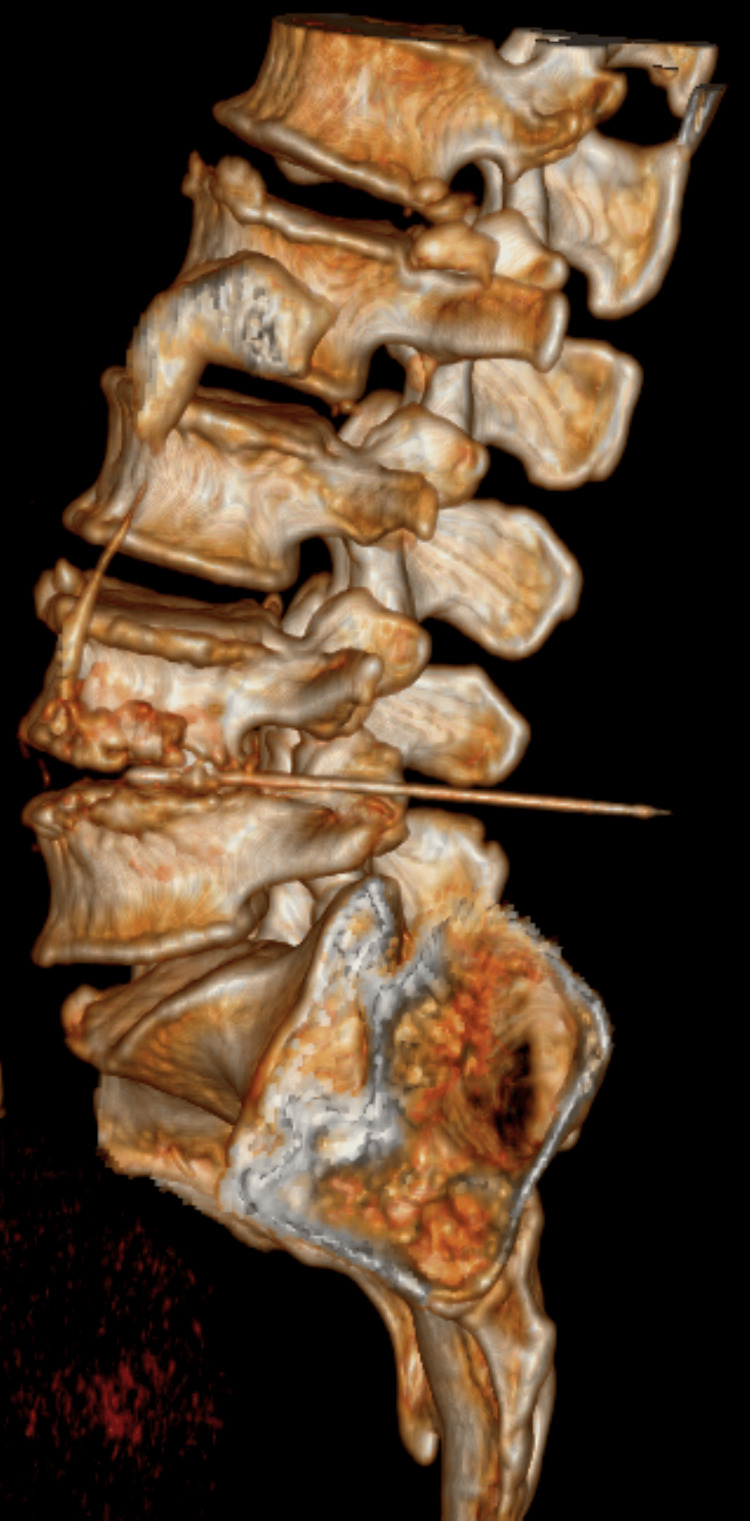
CT of the lumbar spine with 3D sagittal reconstruction, showing CT-guided percutaneous biopsy of the intervertebral disc (L4-L5), performed using a Tru-Cut needle with a coaxial system. CT, computed tomography; 3D, three-dimensional.
